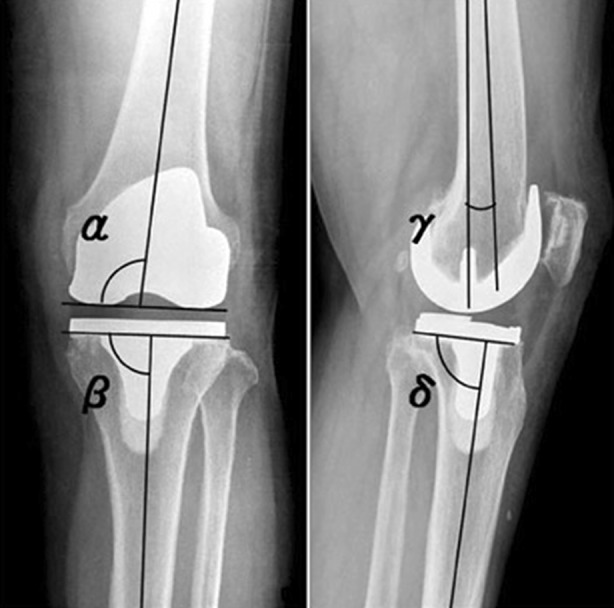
Femoral angle (α) and slope (γ), and tibial angle (β) and slope (δ) as measured on an anterior-posterior radiograph and on a lateral radiograph
